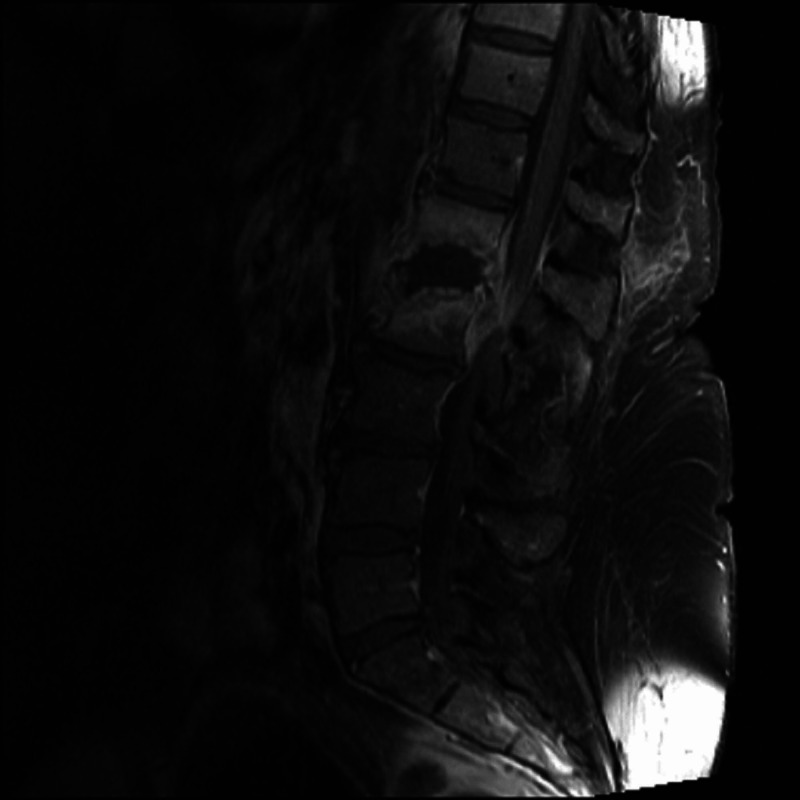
MRI revealed discitis and osteomyelitis at L1-L2 with spinal epidural abscess causing severe compression of the cauda equina nerve roots.
